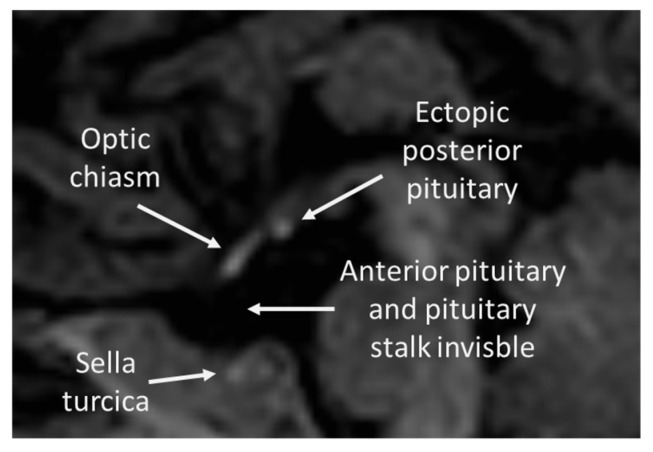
Typical features of PSIS in Patient’s 1 MRI (sagittal plain).
